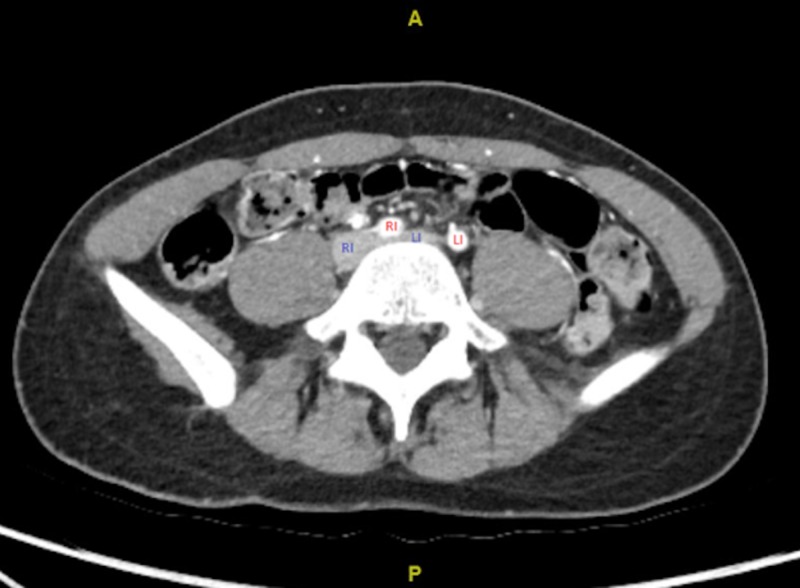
CTA aorta with runoff to lower extremity noting flattening of the left common iliac vein (blue LI) by the right iliac artery (red RI). Right common iliac vein (blue RI) and left iliac artery (red LI) also noted.
